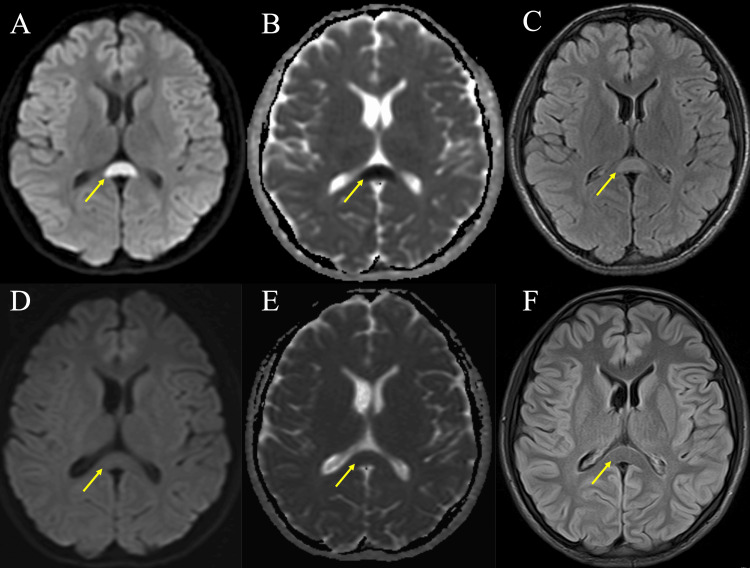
Case 4: MRI findings of CLOCCs (A) DWI shows a high-signal-intensity lesion in the splenium of the corpus callosum (yellow arrow). (B) The ADC map shows low signal intensity, consistent with cytotoxic edema (yellow arrow). (C) FLAIR imaging shows high signal intensity in the corpus callosum (yellow arrow). (D-F) Follow-up MRI shows complete resolution of the corpus callosum abnormalities. ADC, apparent diffusion coefficient; CLOCCs, cytotoxic lesions of the corpus callosum; DWI, diffusion-weighted imaging; FLAIR, fluid-attenuated inversion recovery
